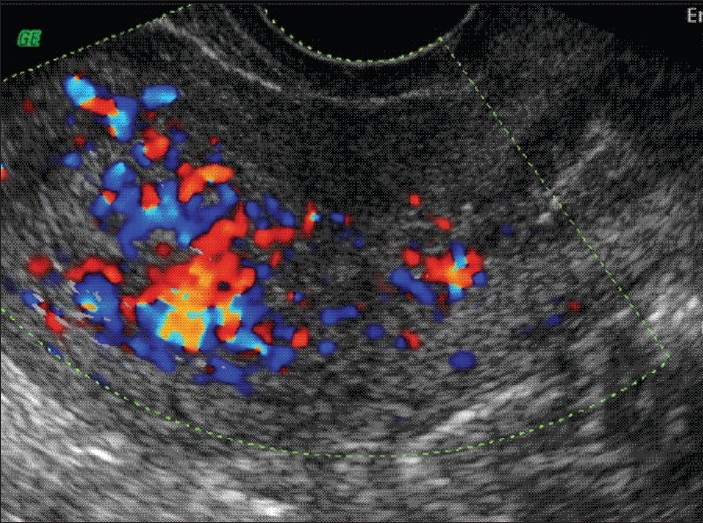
Doppler showing increased endometrial vascularity
